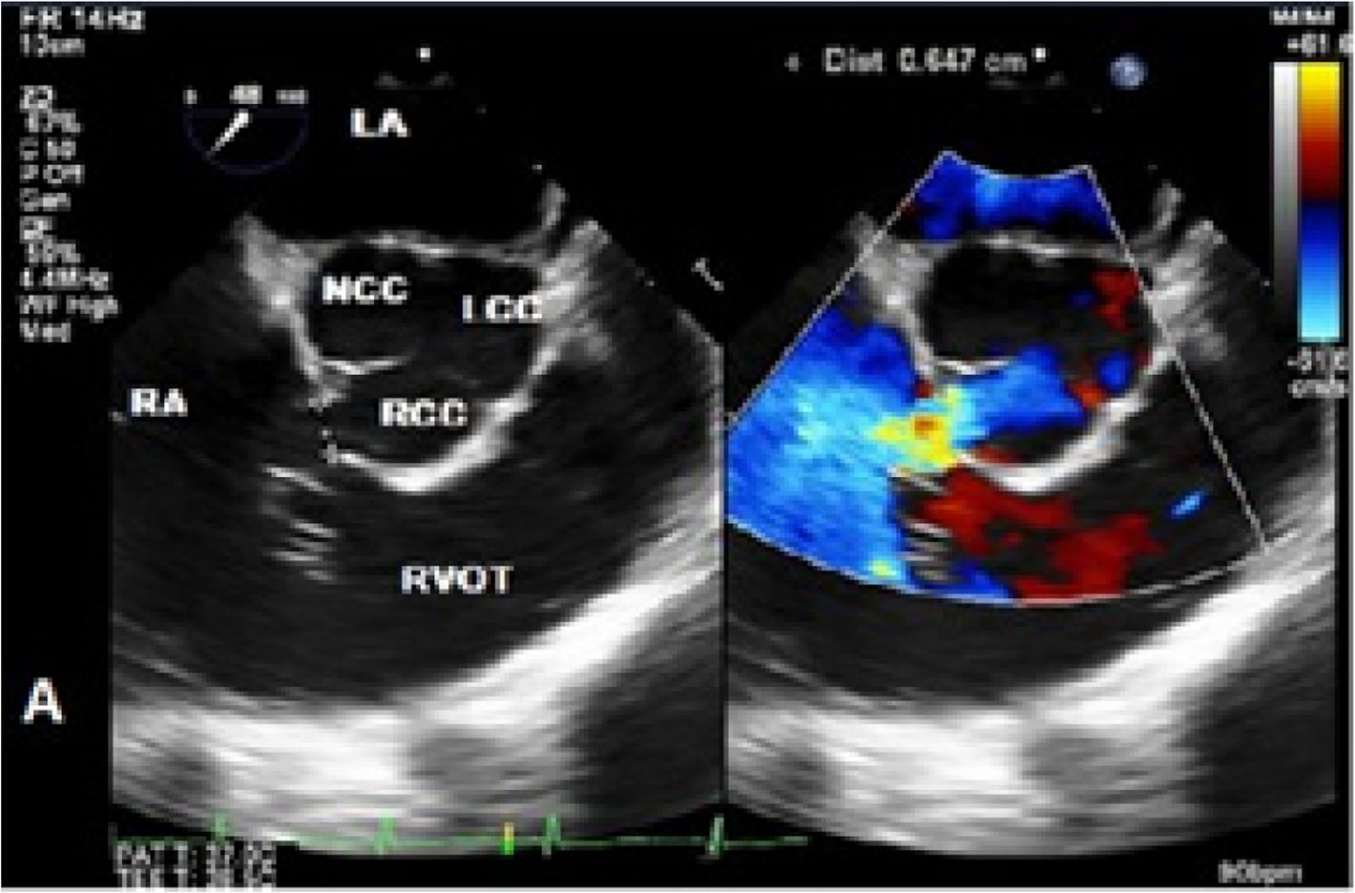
Two-dimensional transoesophageal echocardiography in mid-oesophagus short axis with colour compare view showing a left-to-right shunt from the right sinus of Valsalva to the right atrium. RVOT, right ventricular outflow tract; RV, right ventricle; RA, right atrium; LA, left atrium; RCC, right coronary cusp; NCC, non-coronary cusp; LCC, left coronary cusp
